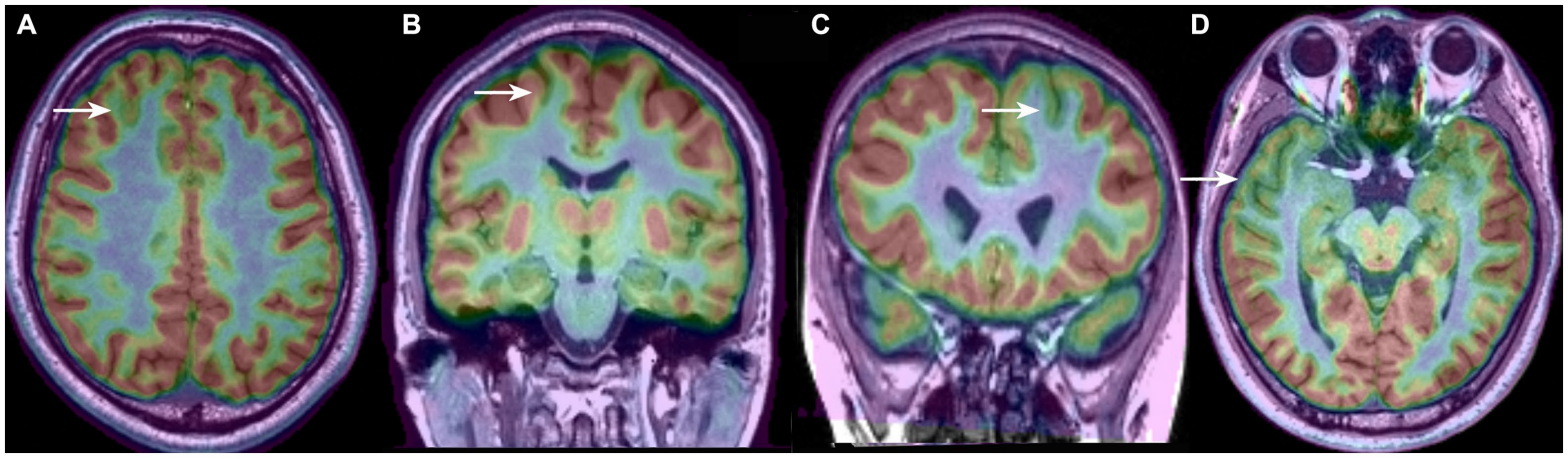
Four types of PET-MRI hypometabolic patterns of FCD: (A) bottom of sulcus hypometabolism (BOSH); (B) single island of sulcus hypometabolism (SIOS); (C) single gyrus or sulcus hypometabolism (SGOS); (D) and multiple gyri and sulci hypometabolism (MGOS).
